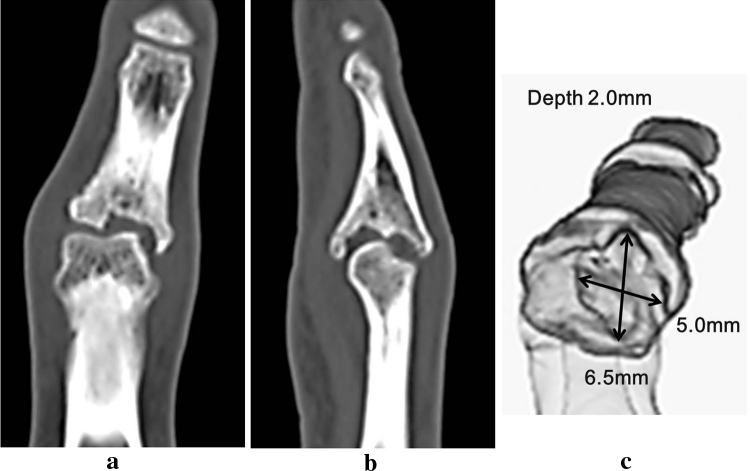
CT images before treatment. a Axial image, b sagittal image, and c 3D image. A bony defect 5.0 × 6.5 × 2.0 mm in size was seen in the articular surface of the PIP joint of the middle phalanx
